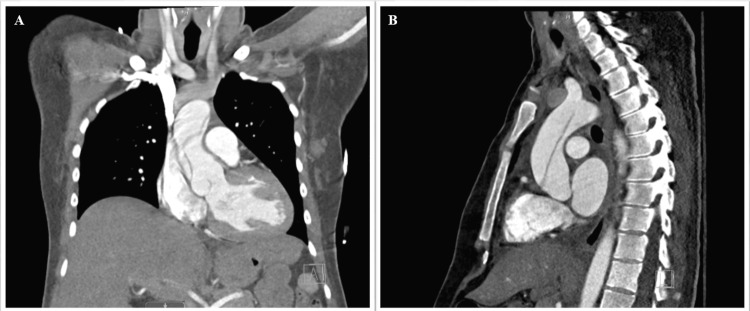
The Patient's Chest CT at Initial Presentation Showing Type A Aortic Dissection Extending to the Aortic Arch in Coronal (a) and Sagittal (B) Views.
